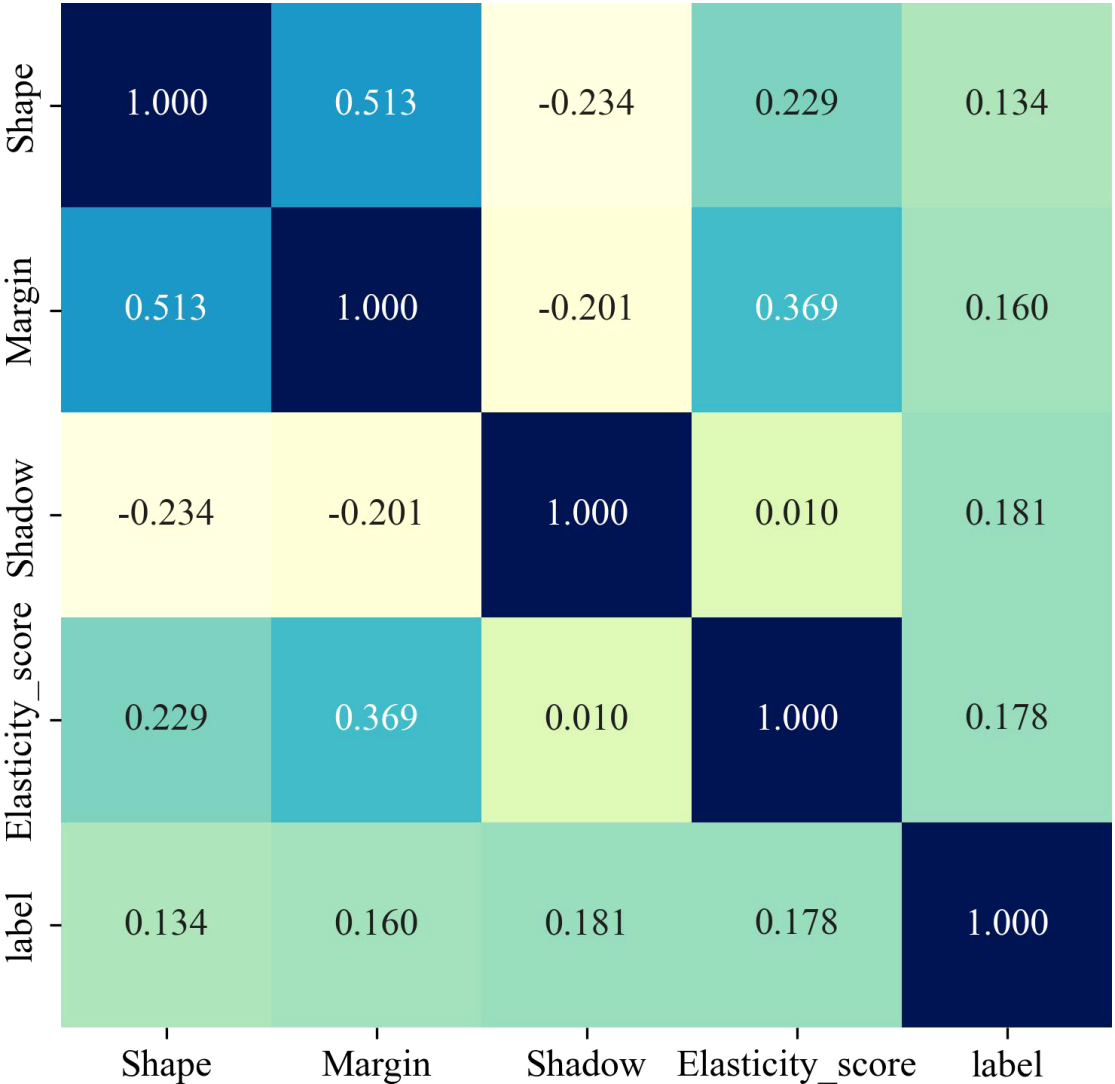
Spearman correlation of each clinical and ultrasound feature.
